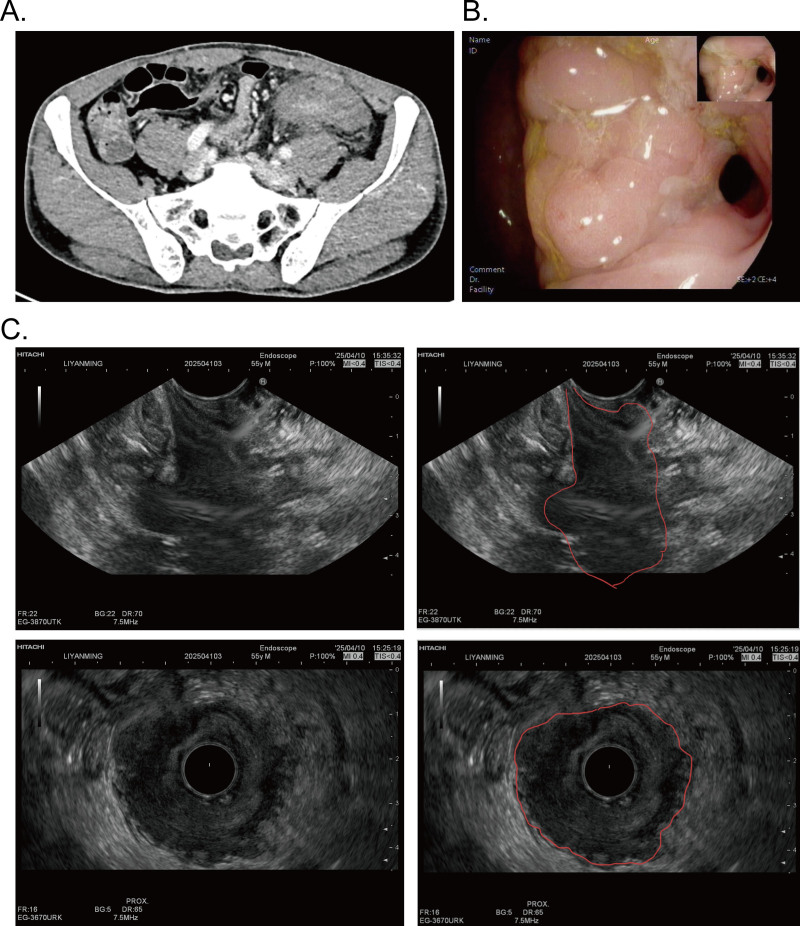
A, In computed tomography imaging, inflammatory myofibroblastic tumors (IMT) presented as well-defined soft tissue masses with heterogeneous enhancement. B, Endoscopically, IMT presented as a submucosal protuberant lesion with an intact overlying mucosa. C, Under EUS, IMT appeared as a heterogeneous hypoechoic mass with relatively well-defined boundaries.
